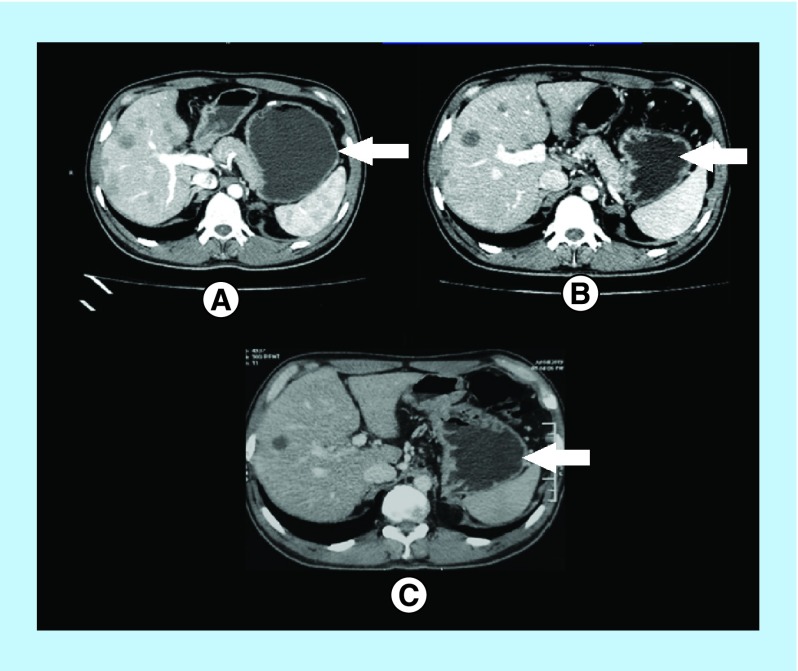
Computed tomography scan showing the largest lesion: (A) before start of the treatment; (B) post 3 cycles; (C) post 17 cycles.
